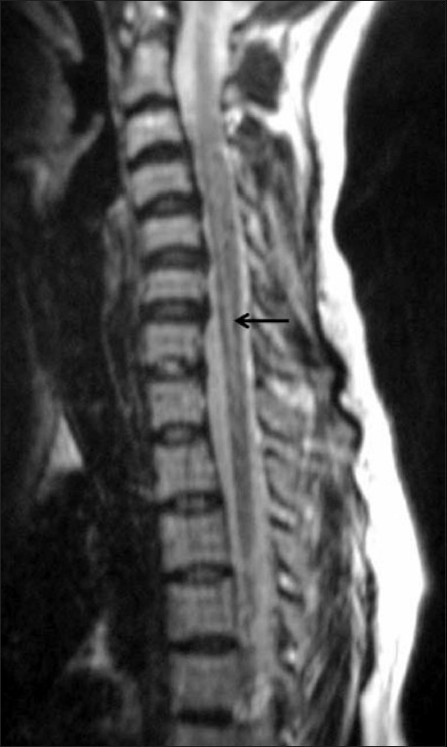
Sagittal T2W MRI image of the cervical spine shows central hyperintensity (arrow) extending from C3 to C7
